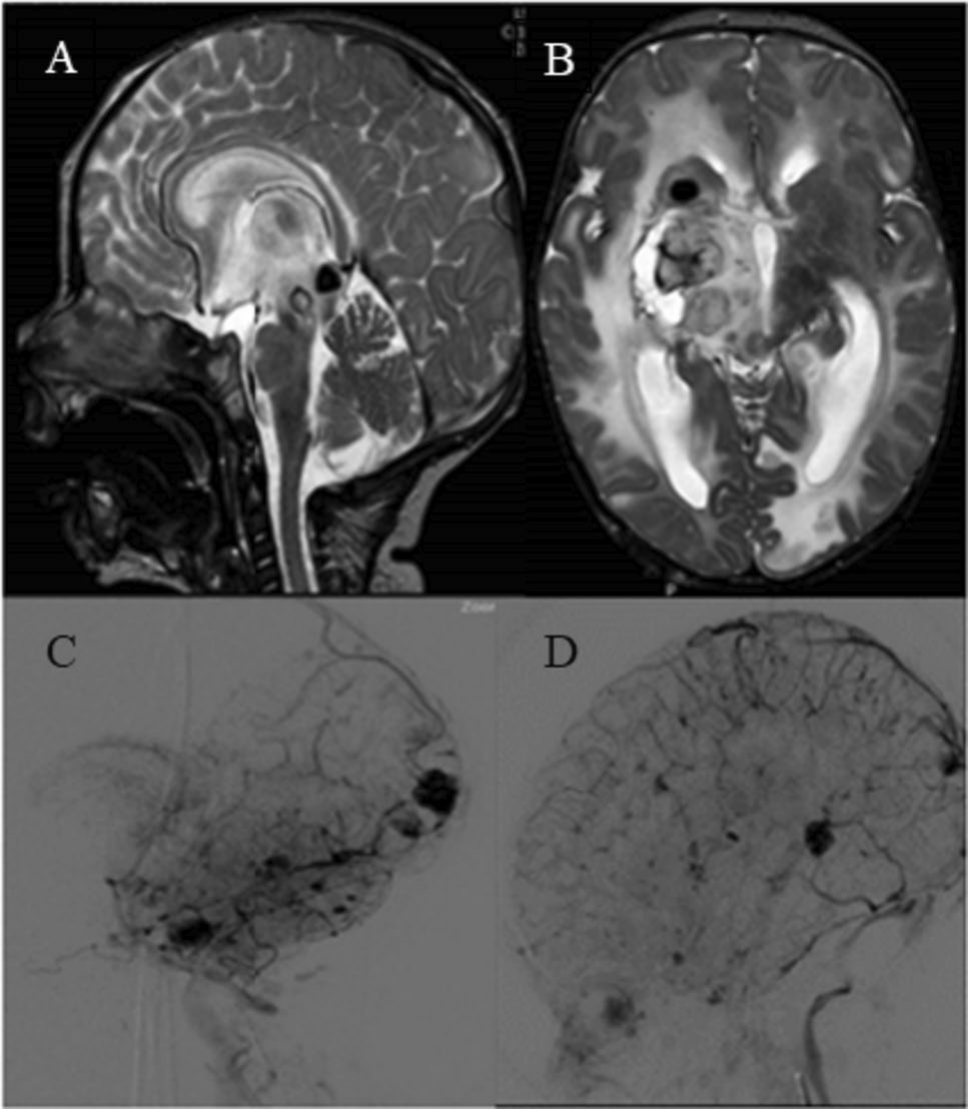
A, B At initial presentation in sagittal and axial T2-weighted images (T2WI) showing acute and multiple (nucleo-capsular right and tectal mesencephalic) ICH (intracerebral hemorrhages) with periventricular brain vasogenic edema of white matter. C, D DSA venous phase excluded arteriovenous malformation (AVM) and arteriovenous fistulas (AVFs)
